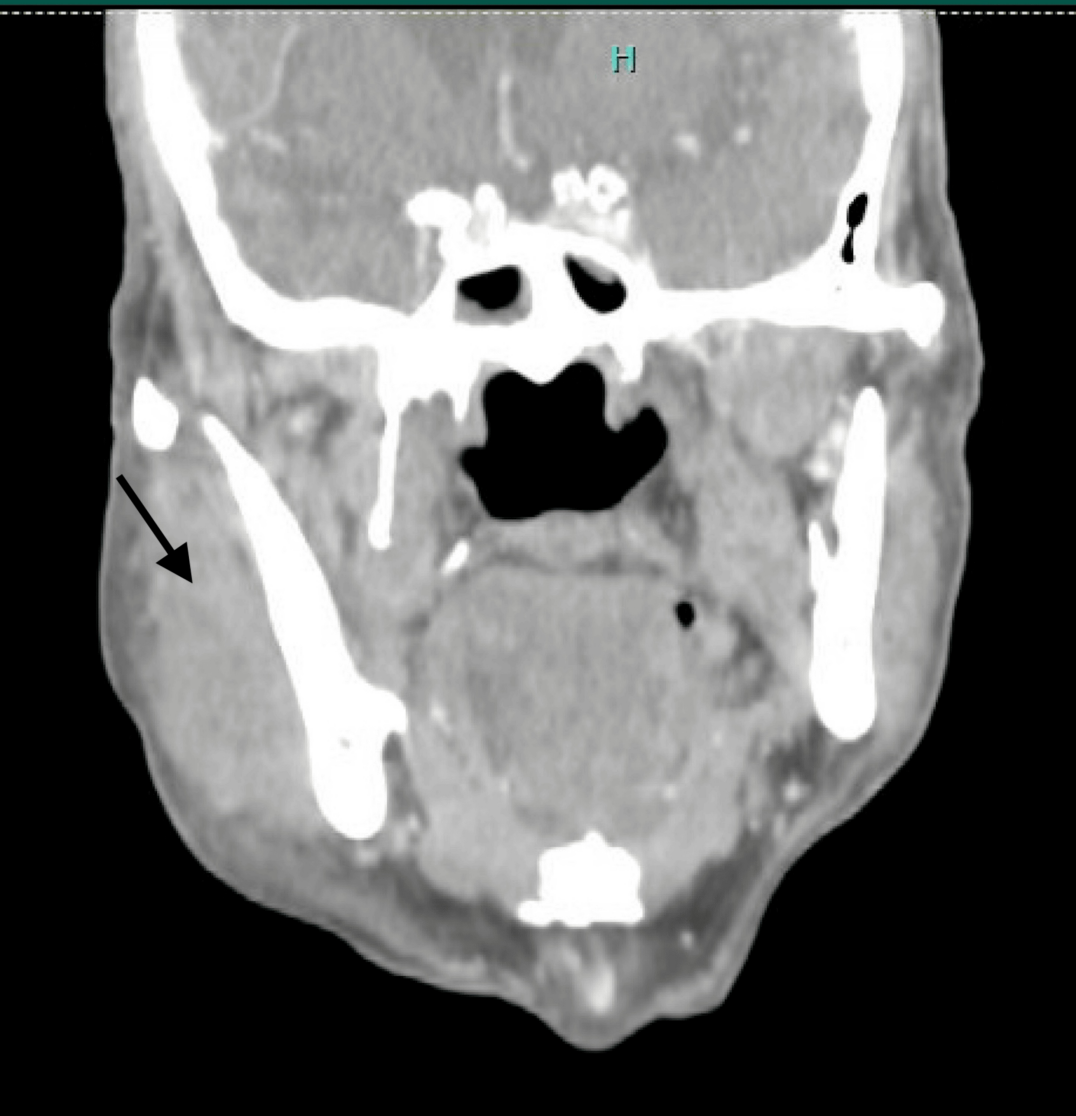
Computed tomography of the soft tissue neck demonstrating a right masseter hematoma (black arrow).
